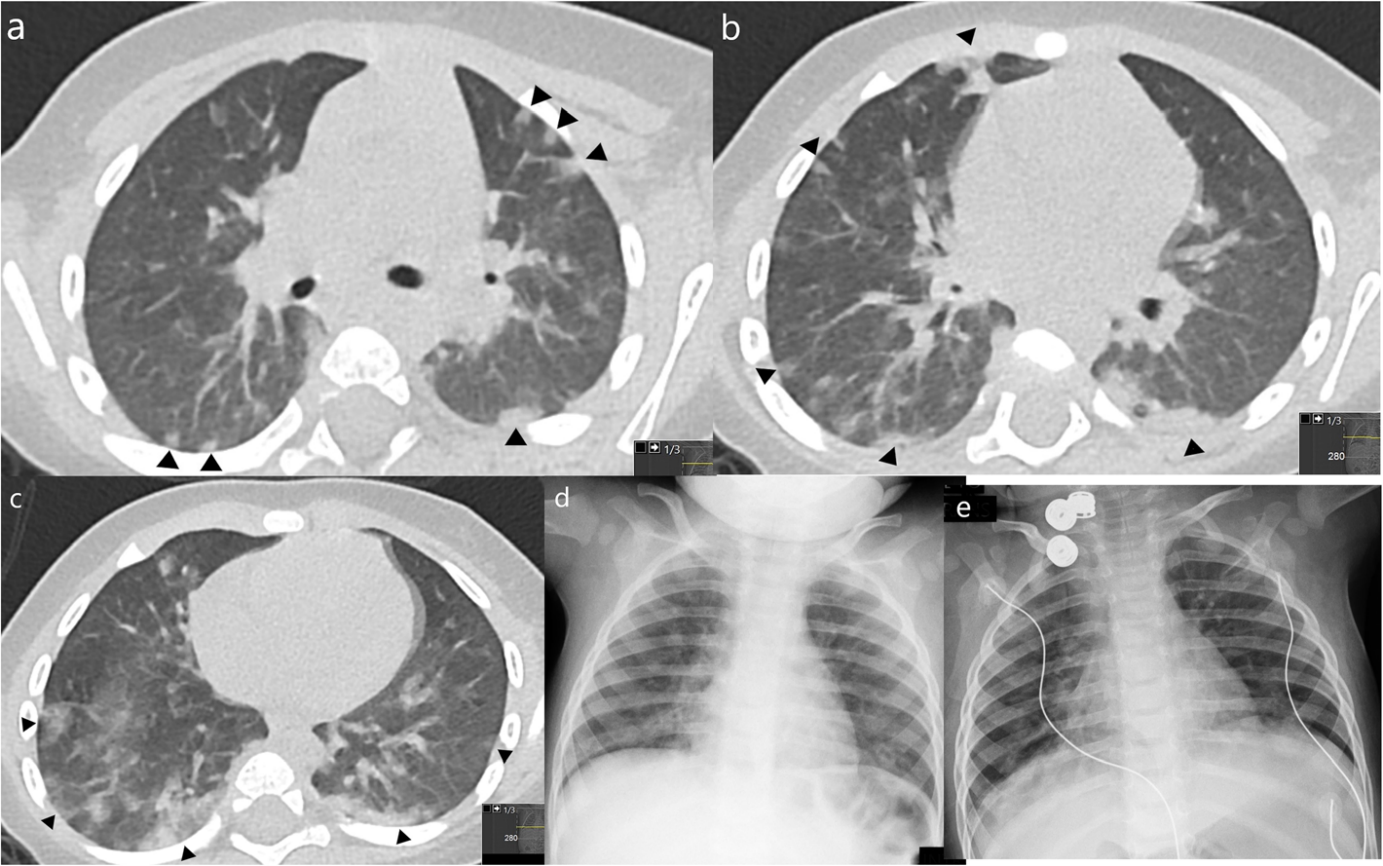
Typical findings in a 10-month-old infant. Patchy consolidations and GGO in the upper and lower lobes predominantly seen in the subpleural pulmonary parenchyma (a–c). Some of these opacities corresponded to an halo sign. Chest X-ray performed the day before the CT (d) and 4 days after (e) shows partial resolution of the subtle bilateral opacities, typical for COVID-19
